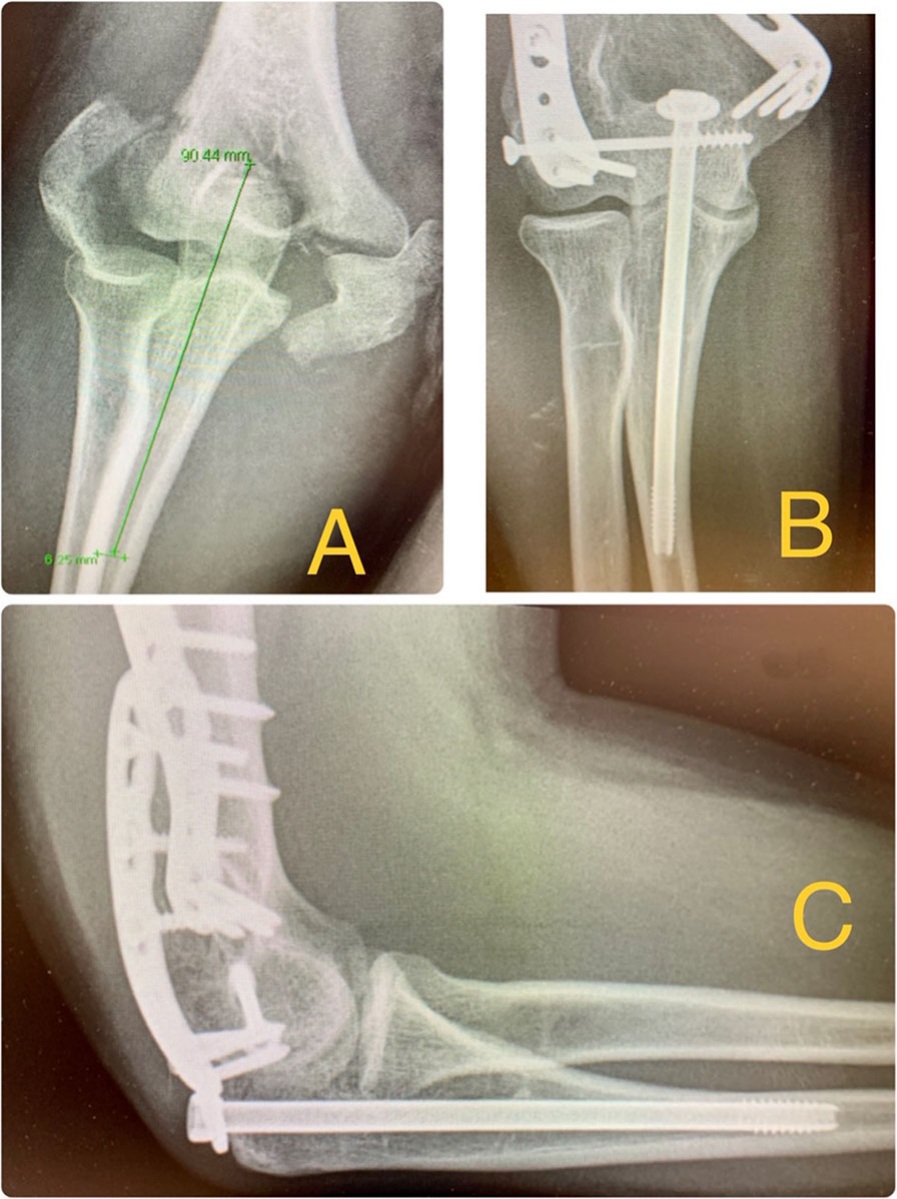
63 year old man who presented a conminuted supraintercondylear fracture of the humerus. Osteosynthesis of the fracture with two plates and a 6.5 mm thick and 110 mm long screw with washer for the olecranon osteotomy. a AP X-ray with the mesured ulna diaphysis diameter (6′25 mm) at 90 mm to the tip of the olecranon. b AP X-ray after 3 months where the consolidation of the osteotomy can be appreciated, the screw is long enough to achieve a good grip in the cortex of the ulnar diaphysis to get enough compression and stability. The medial deviation at the union of the medial and proximal 1/3 third (9–11 cm from the tip of the olecranon) can be observed in this X ray. This fact of the anatomy of the ulna contributes to the screw clamping. c Lateral X-ray after 3 months where the consolidation of the osteotomy can be appreciated, the screw is long enough to achieve a good grip in the cortex of the ulnar diaphysis to get enough compression and stability. The anterior deviation at the union of the medial and proximal 1/3 third (9–11 cm from the tip of the olecranon) can be observed in this X-ray. This fact of the anatomy of the ulna contributes to the screw clamping
